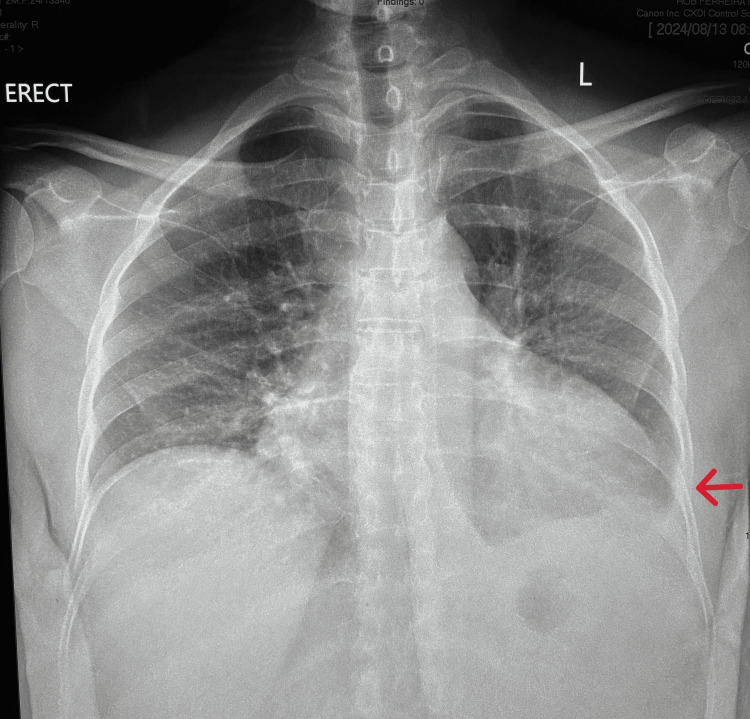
Anteroposterior chest roentgenogram demonstrating pleural effusion in left hemithorax (red arrow)
